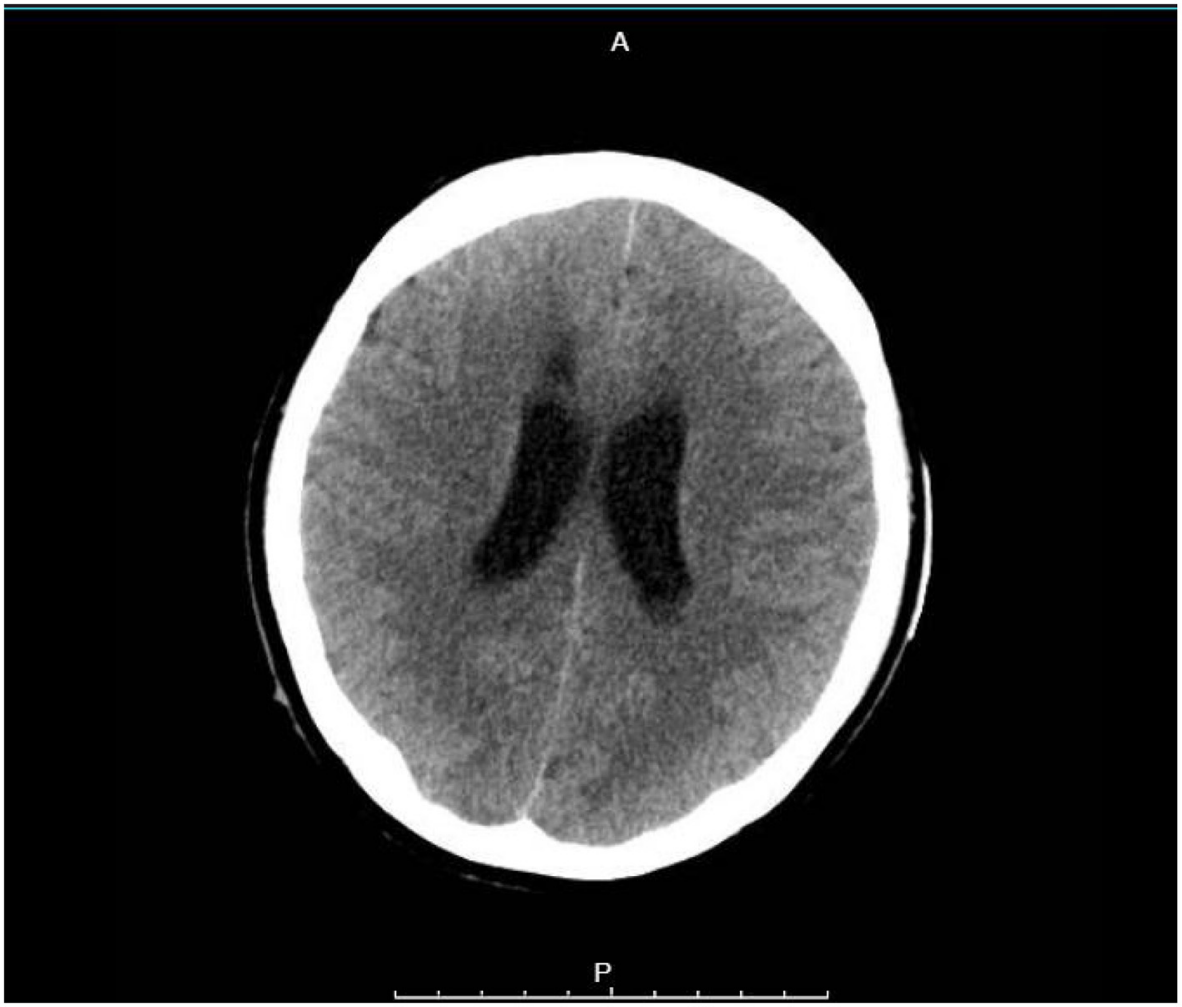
Follow-up non-contrast axial CT scan obtained on the sixth postoperative day after endoscopic evacuation, demonstrating significant clearance of the intraventricular hematoma with restored ventricular anatomy and cerebrospinal fluid pathways.
